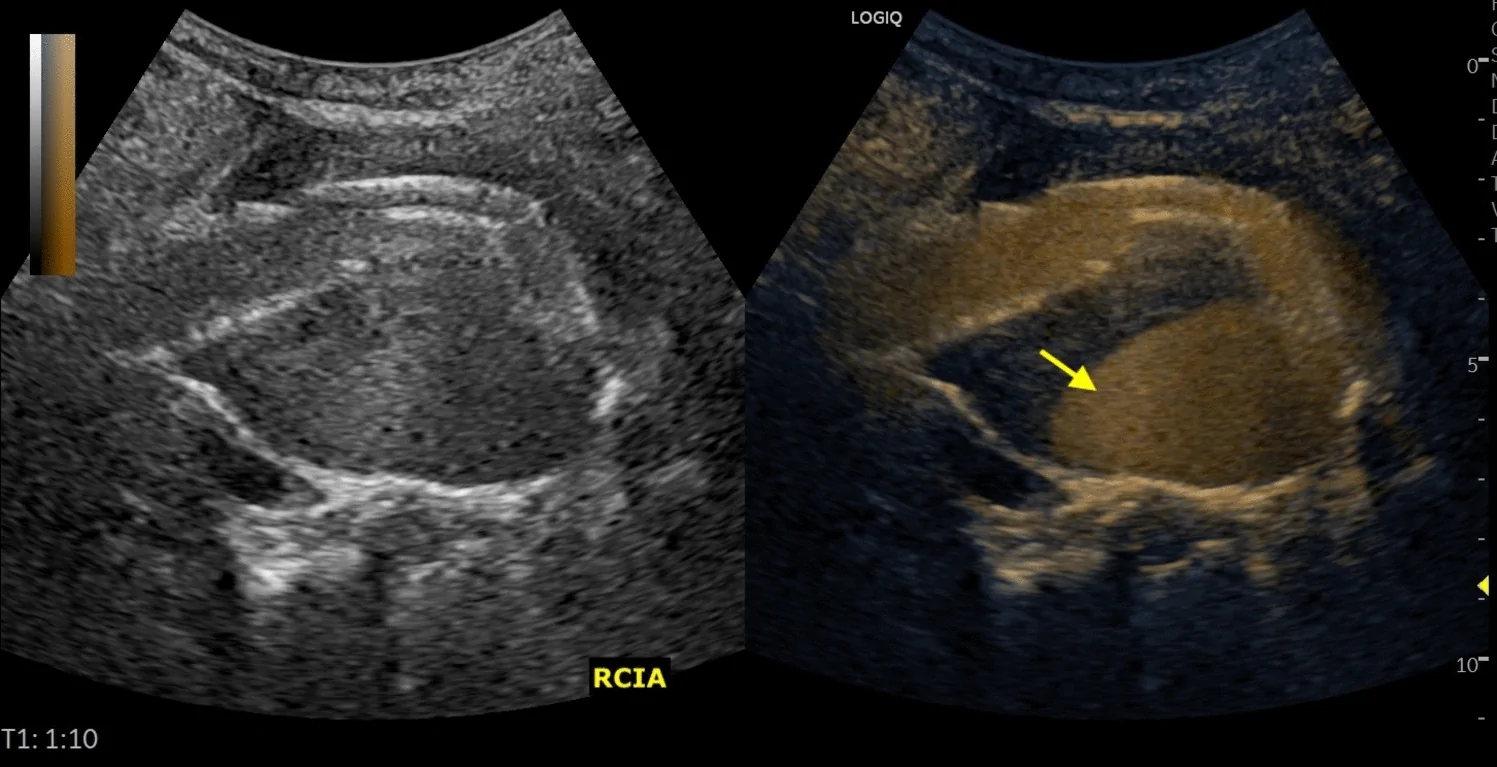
Contrast-enhanced ultrasound (CEUS) of endoleak detection after endovascular aneurysm repair (EVAR). Split-screen showing B-mode ultrasound (left) and contrast-specific low–mechanical index imaging (right) after intravenous sulfur hexafluoride microbubbles (SonoVue^®^). Enhancement within the aneurysm sac (arrow) indicates a type III endoleak not detected on colour Doppler ultrasound (DUS)
